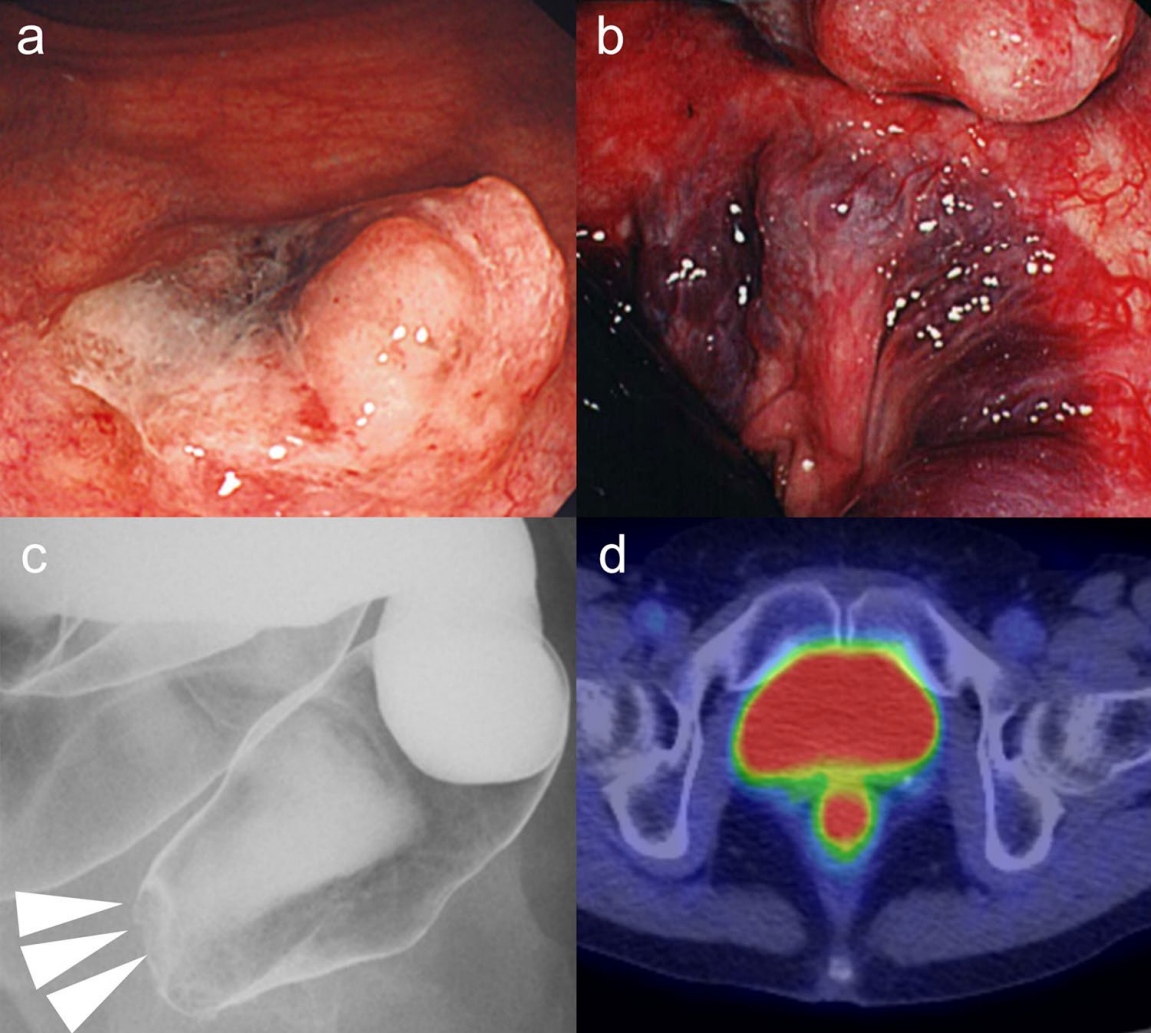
Results of the examination before the first surgical treatment. a Colonoscopy revealed a 20 × 13 mm lesion in the lower rectum (4 cm from the anal verge) with a blackish depression in the center. b Colonoscopy, revealed a lesion directly above the dentate line. c Gastrointestinal enema showing a contrast defect in the anterior wall of the lower rectum. d PET showed a localized abnormal accumulation of Max SUV: 6.4–8.6 in the lower rectum
